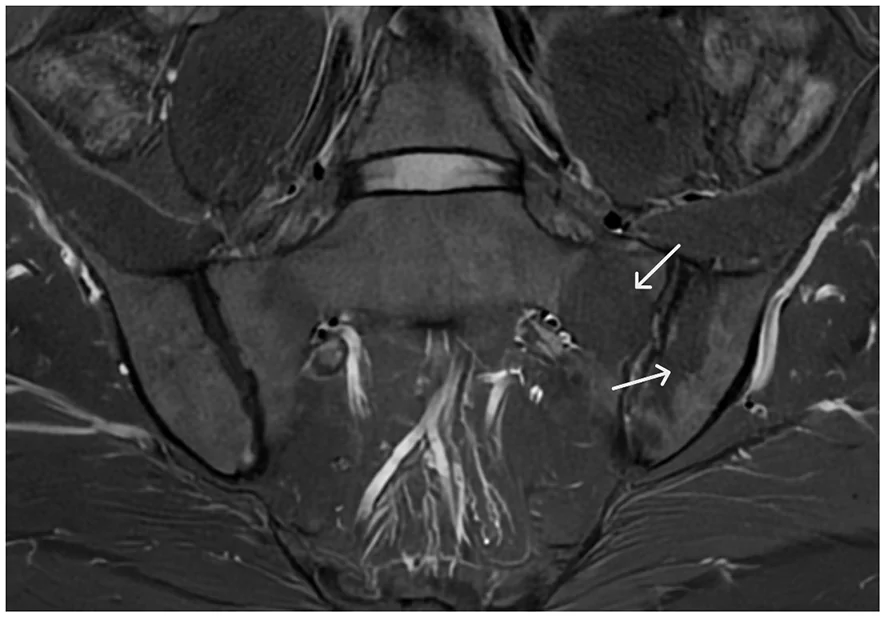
Follow-up semicoronal short tau inversion recovery (STIR) MRI of the sacroiliac joints obtained after 6 months of adalimumab therapy and in the same plane as the baseline study. White arrows indicate the corresponding anatomical sites of the previously demonstrated inflammatory lesions. Compared with baseline, there is marked interval reduction in subchondral bone marrow edema, with no definite residual focal fluid-sensitive sacroiliac signal abnormality on the displayed sequence. Bone marrow edema was not formally scored using the SPARCC system, and the comparison with baseline is therefore qualitative.
